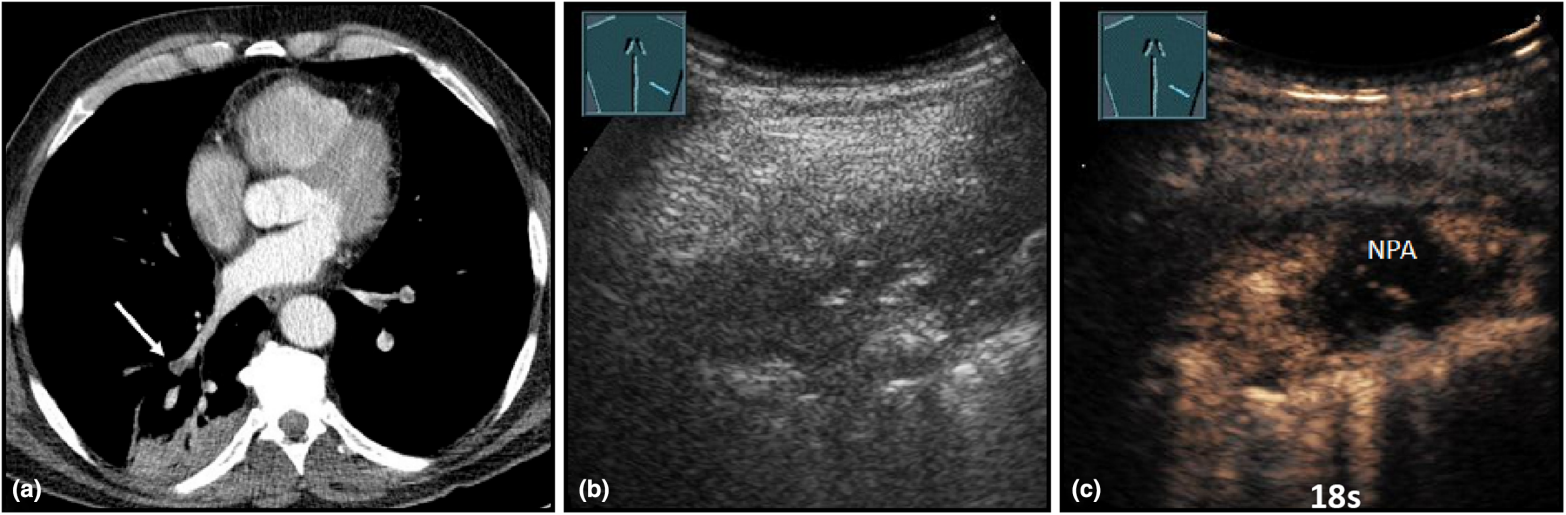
A 56‐year‐old patient was in intensive care with fever, shortness of breath, evidence of deep vein thrombosis and pulmonary artery embolism following non‐invasive ventilation, and known cardiomyopathy. Visualisation of a right‐sided, dorsal, peripheral consolidation on computed tomography (courtesy of Prof. Dr. Andreas H. Mahnken, Department of Radiology, University Hospital Marburg) (a), with central, pulmonary artery embolism (arrow); B‐mode ultrasound (b) shows a hypoechoic, inhomogeneous consolidation with air reflexes; contrast‐enhanced ultrasound (c) shows inhomogeneous enhancement with non‐perfused peripheral areas (NPA) after 18 s, indicating the presence of infarct pneumonia.
